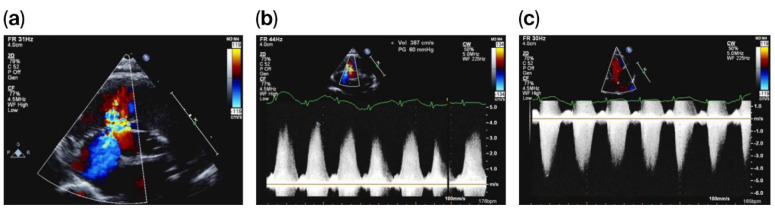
PMVSD with adjacent eccentric tricuspid regurgitation (a), with VSD Doppler (b) and tricuspid regurgitation Doppler (c).
